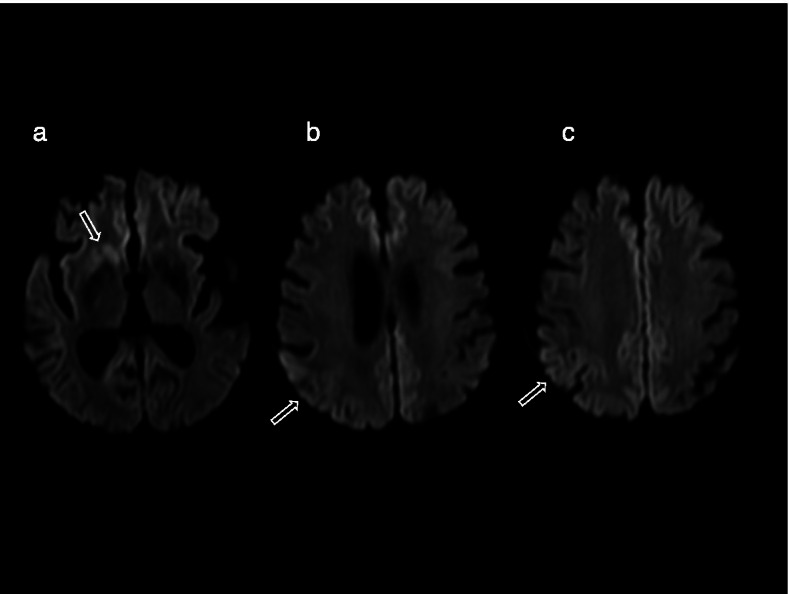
Diffusion-weighted magnetic resonance imaging from 4 months after the onset of memory impairment. The magnetic resonance images show hyperintense signals in the right lenticular and caudate nuclei (a) and right parietal cortex (b, c)
